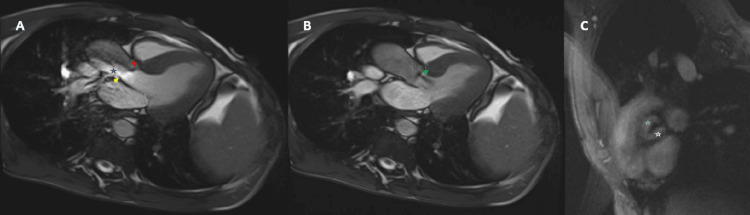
Cardiac MRI Cardiac MRI showing A) three-chamber view in long axis during systole showing severe bicuspid aortic stenosis with a large anterior leaflet (red arrow), smaller posterior leaflet (yellow arrow), and eccentric jet of blood (blue star) across the valve B) during diastole showing mild aortic regurgitation jet (green arrow) C) short-axis view showing bicuspid aortic valve with large anterior (green star) and small posterior leaflet (white star).
